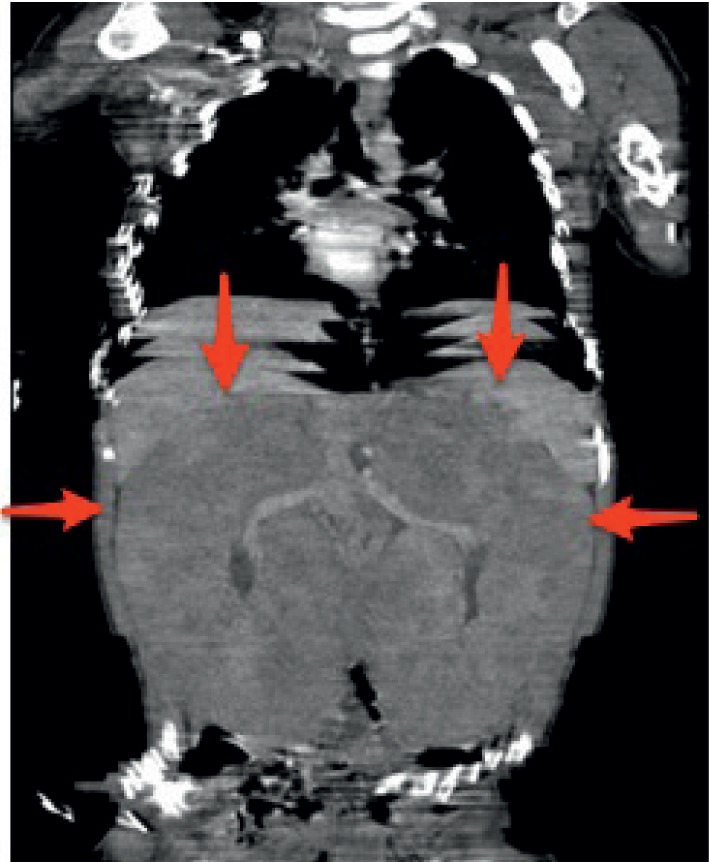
Coronal CT image shows bilaterally enlarged kidneys with decreased enhancement. Enhanced patchy parts are normal renal parenchyma; note the small size of normal parenchyma. Typical for diffuse lymphoma involvement, bilateral kidneys retain their normal bean-like shape.
